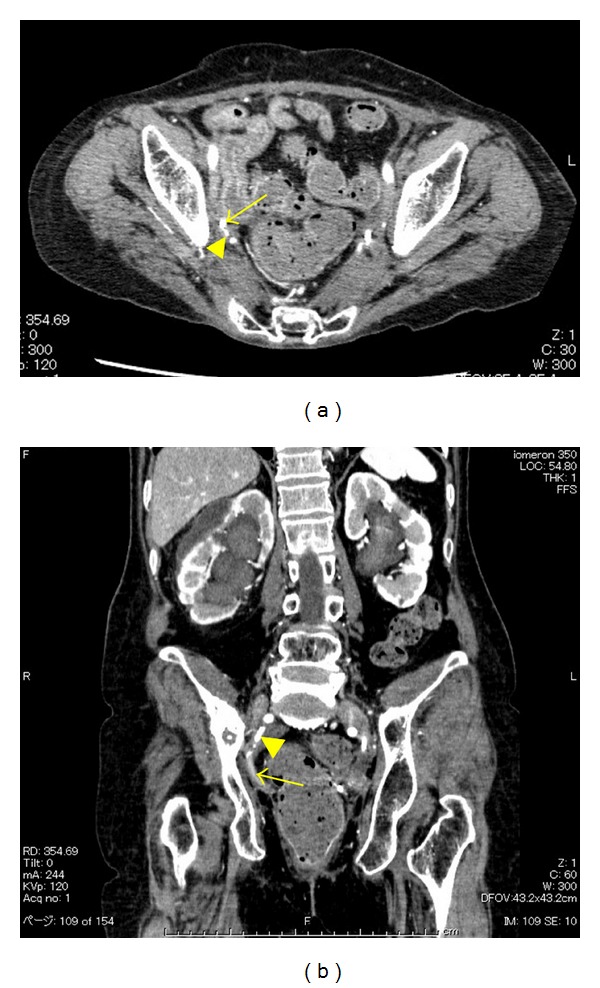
(a) Enhanced computed tomography scan showing the right ureter (arrow) and the right internal pudendal artery (arrowhead). (b) Three-dimensional computed tomography showing the right ureter (arrow) and the right internal pudendal artery (arrowhead).
